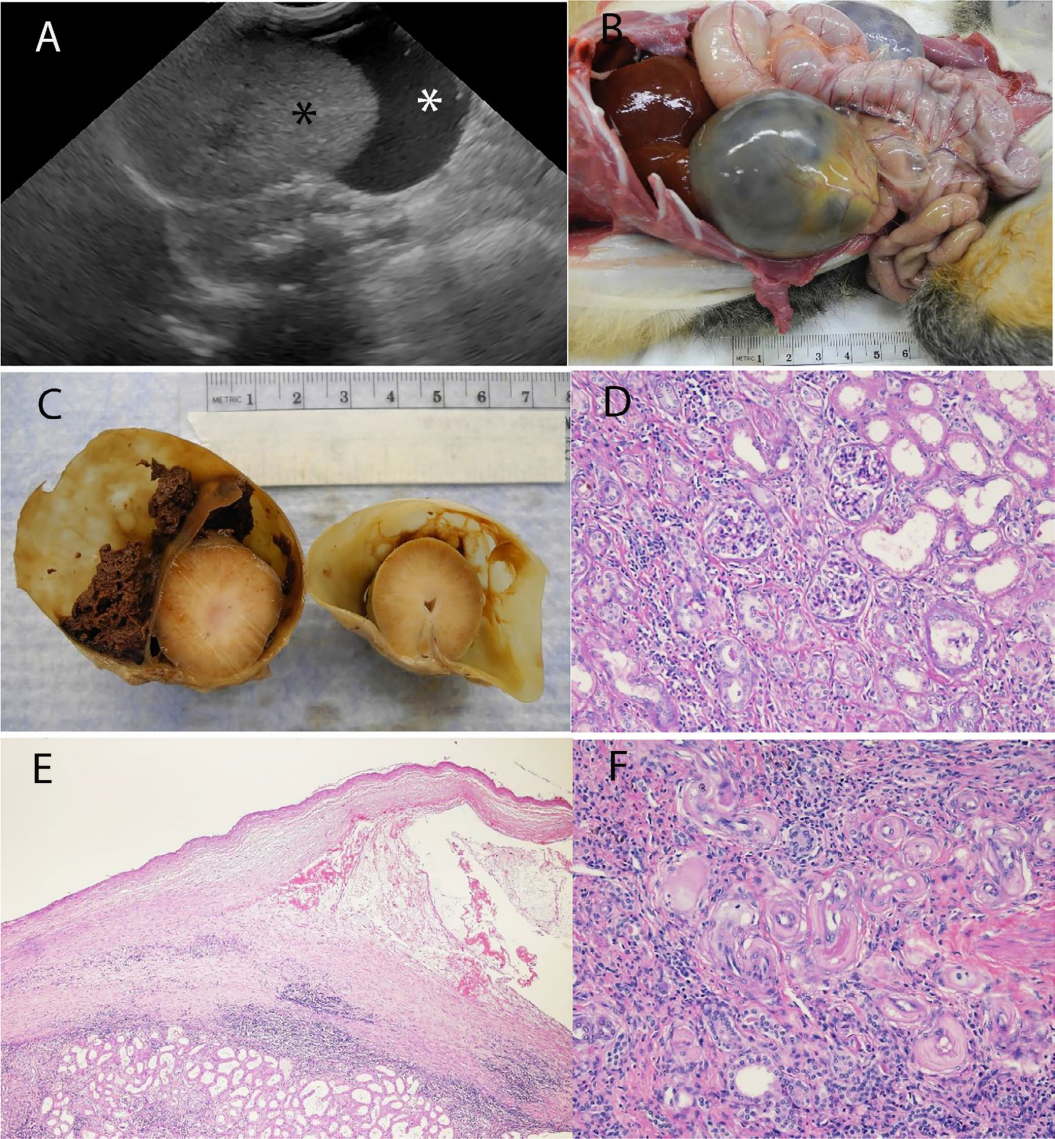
(A) *Aotus nancymai*. Ultrasound image of the right kidney long axis with a perinephric pseudocyst. The kidney is partially surrounded by anechoic fluid (white asterisk). The wall of the perinephric pseudocyst is not clearly visible. The kidney appears normal size but with an increase in the echogenicity of the medulla resulting in a reduction of normal corticomedullary distinction (black asterisk). (B) *Aotus nancymai*. Postmortem image showing the enlarged kidneys. (C) *Aotus nancymai*. Cross section of the kidneys post fixation showing markedly dilated renal capsules. The left kidney contains blood in the subcapsular space. (D) *Aotus nancymai*. Kidney. Image showing renal interstitial fibrosis with nonsuppurative inflammation, glomerular sclerosis, and dilated renal tubules. HE, 200×. (E) *Aotus nancymai*. Kidney. Low power image showing severe inflammation of the renal cortex with separation of the capsule. HE, 100×. (F) *Aotus nancymai*. Kidney. Image showing on cross section several blood vessels with marked hyaline arteriopathy and hyperplastic arteriosclerosis. HE, 200×.
